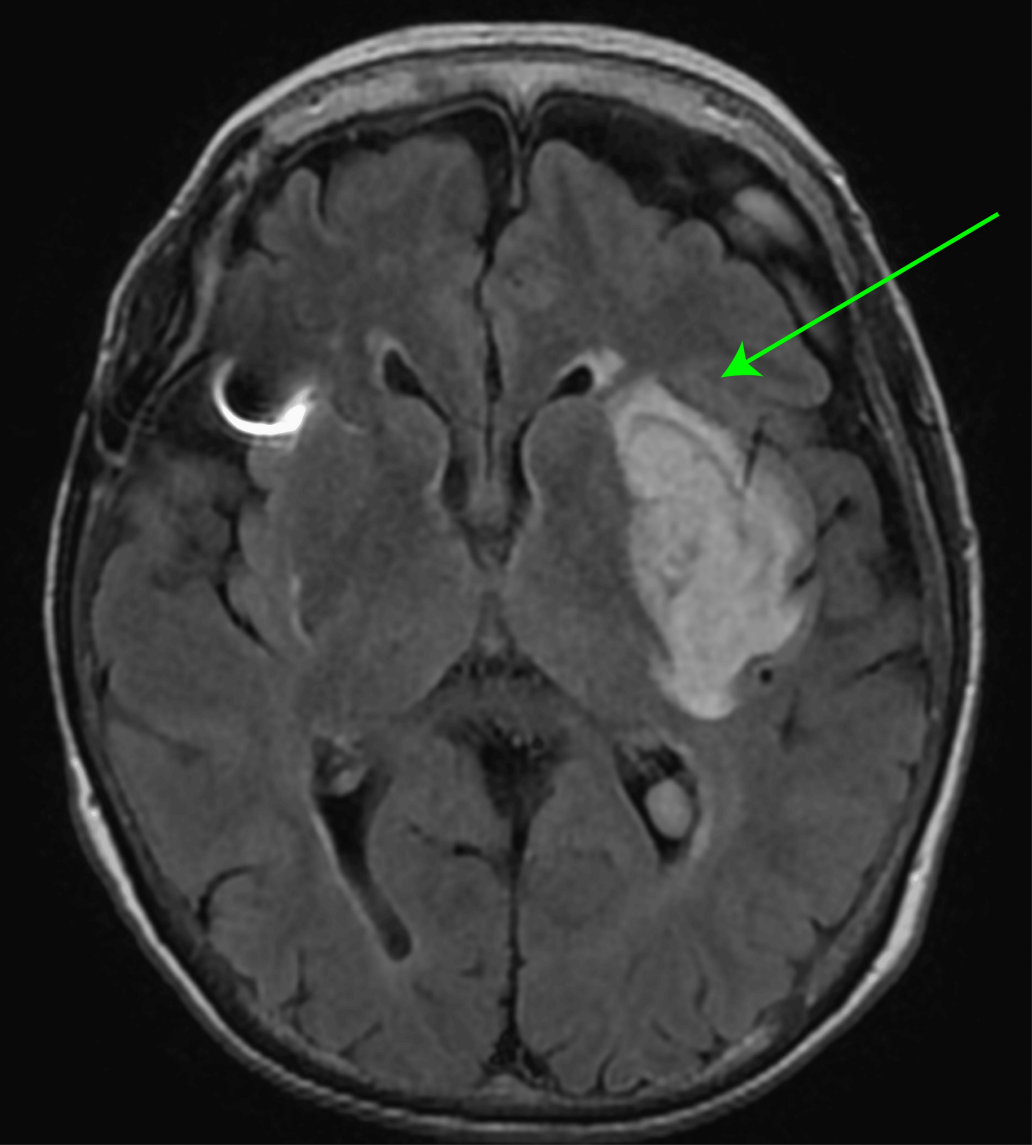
Magnetic resonance image at the age of 72 years old. Magnetic resonance imaging reveals a high-intensity lesion in the left thalamus, indicating a brain hemorrhage (green arrow).
